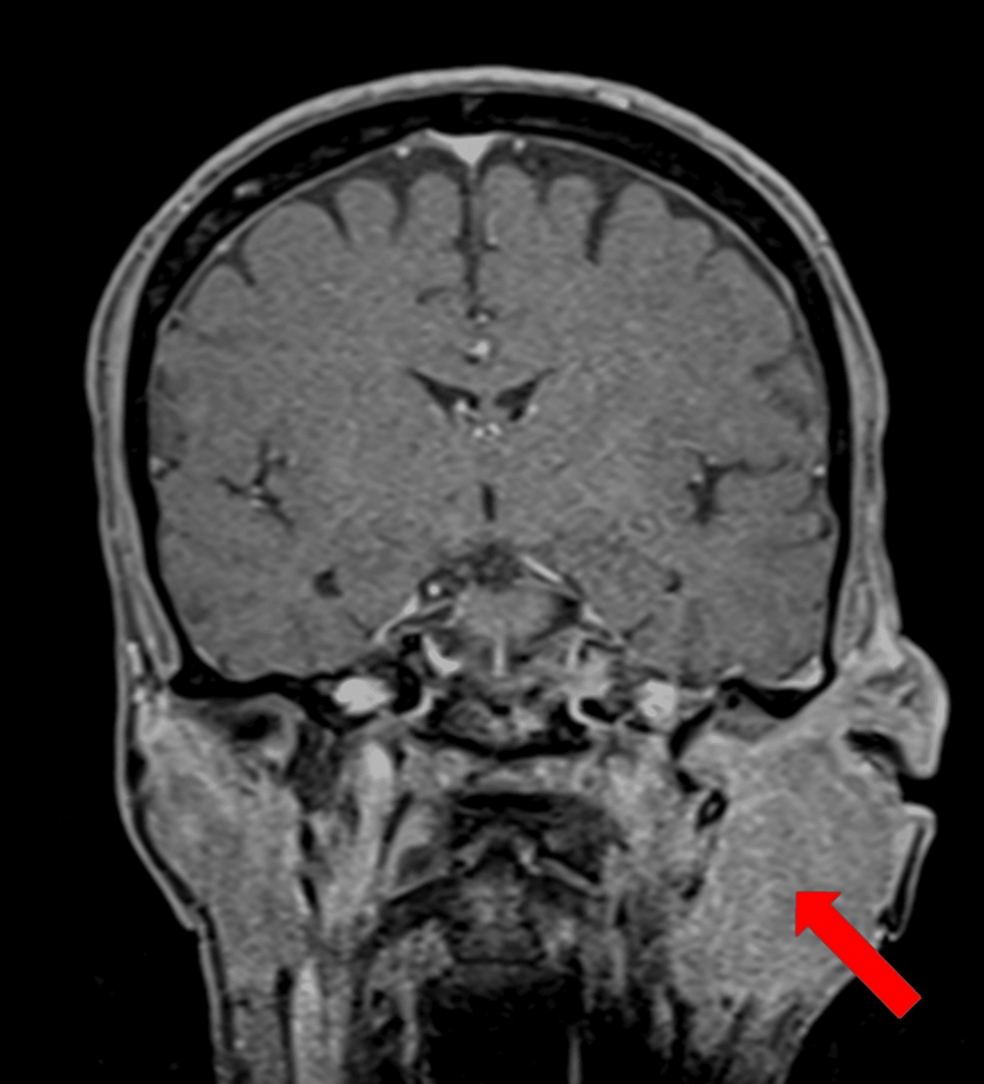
Head MRI. Bulky infiltrative formation with expansion into the parotid gland.
